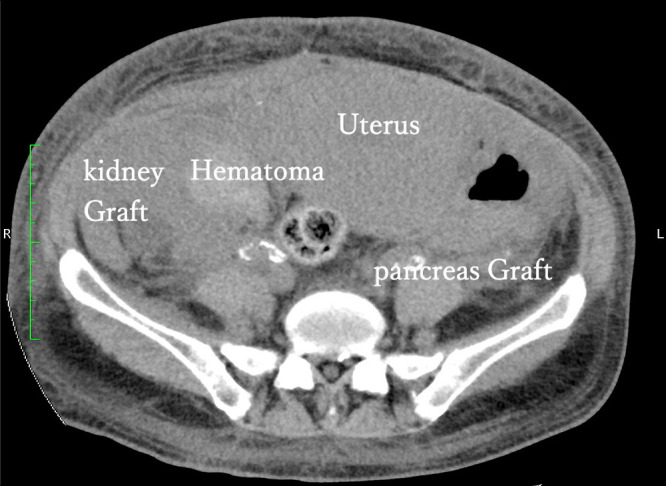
Computed tomography (transverse section) showing a hematoma around the kidney graft. The pancreas graft was intact.
